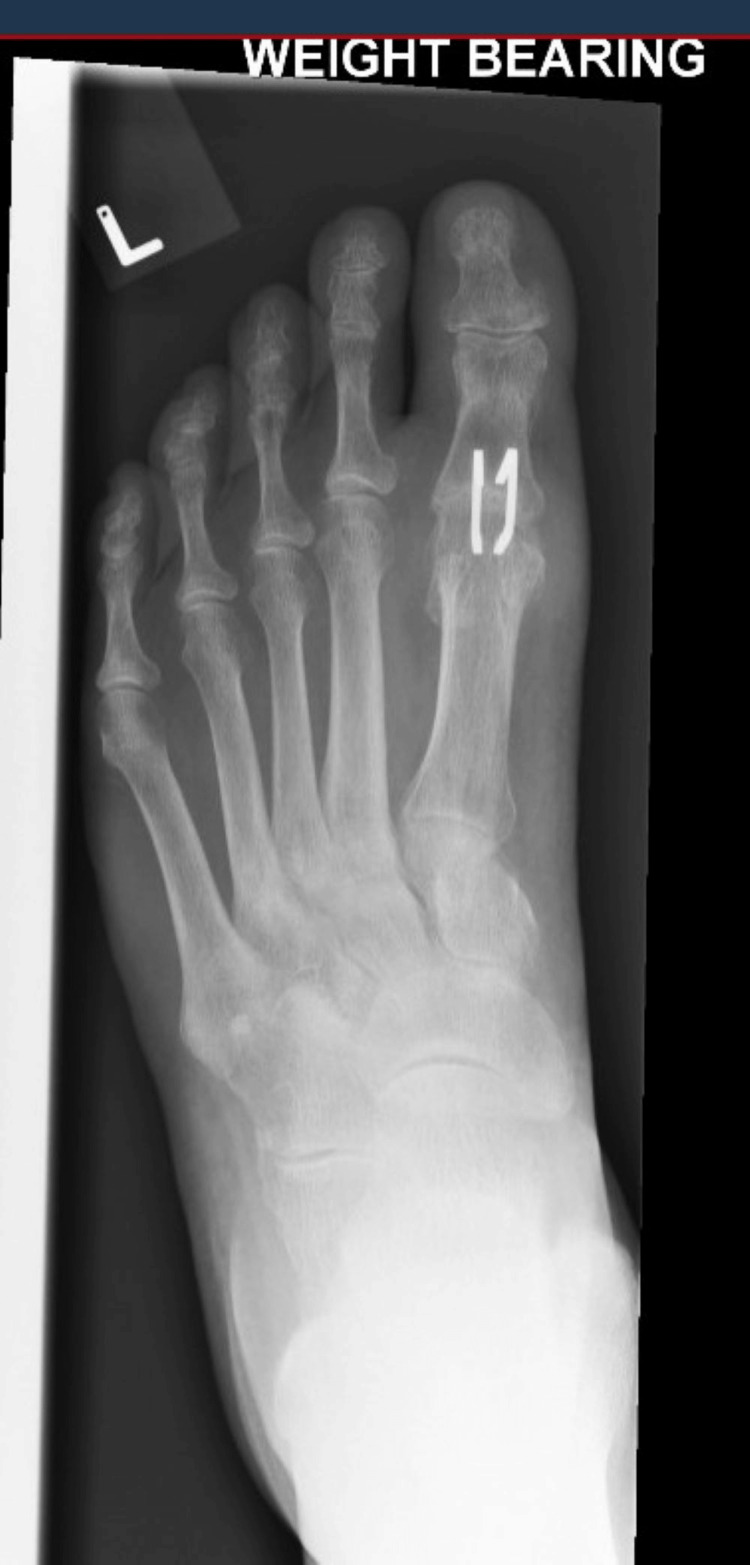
X-ray of left foot taken at three months showing healed fusion site.
